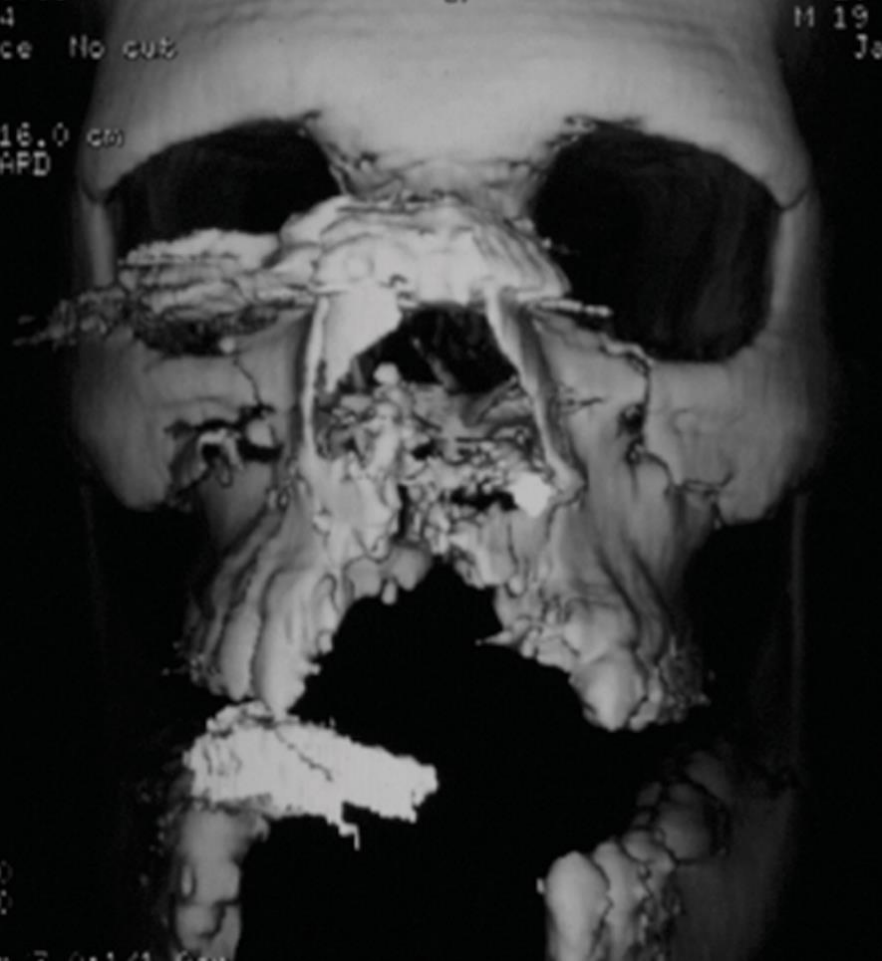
Three dimensional CT scan demonstrating extensive bone
